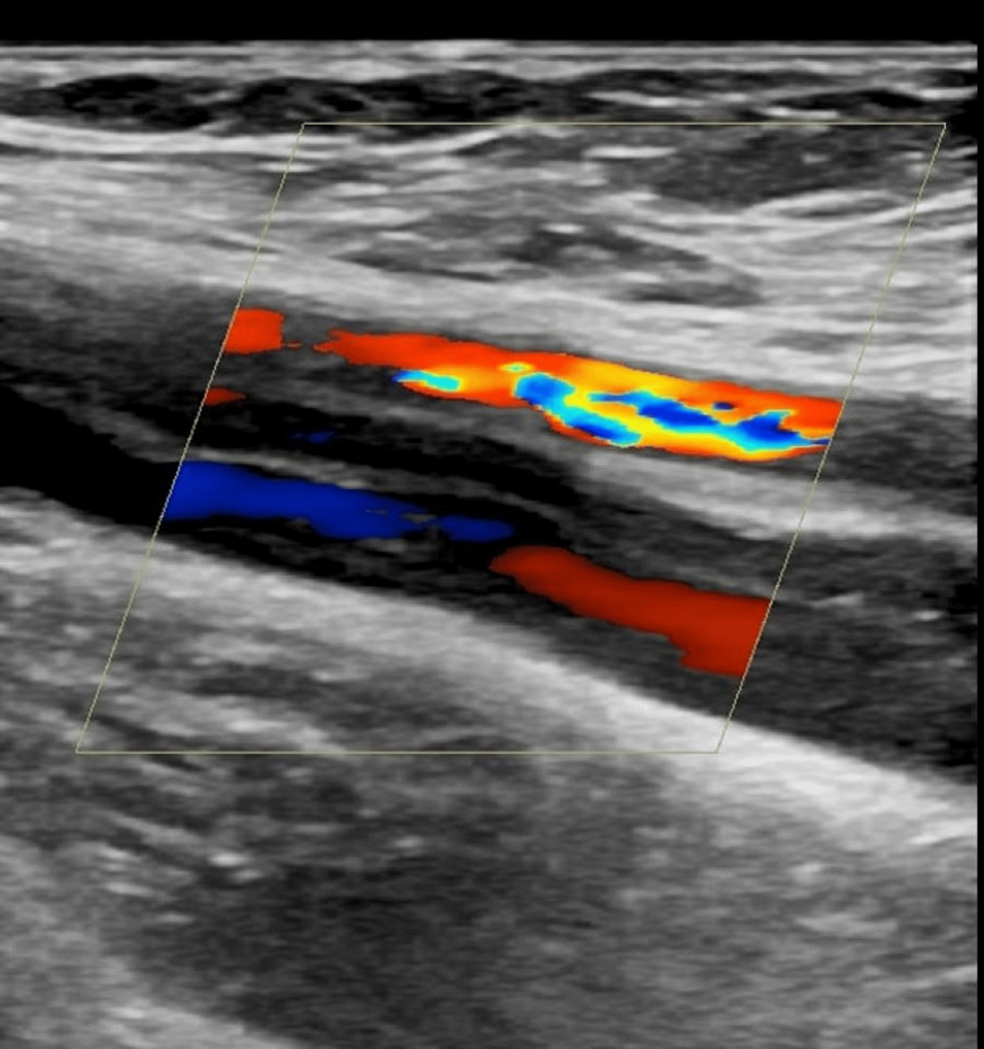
Colour Doppler of the left superficial femoral vein: partially canalized vein after treatment
